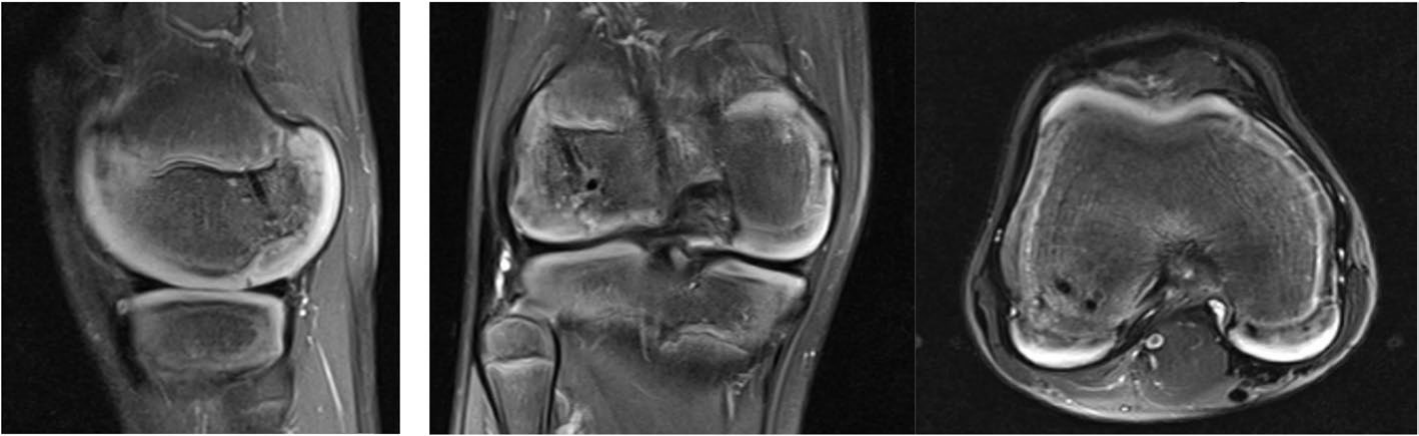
MRI 1 year after surgery demonstrating anatomic fixation and subsequent healing
